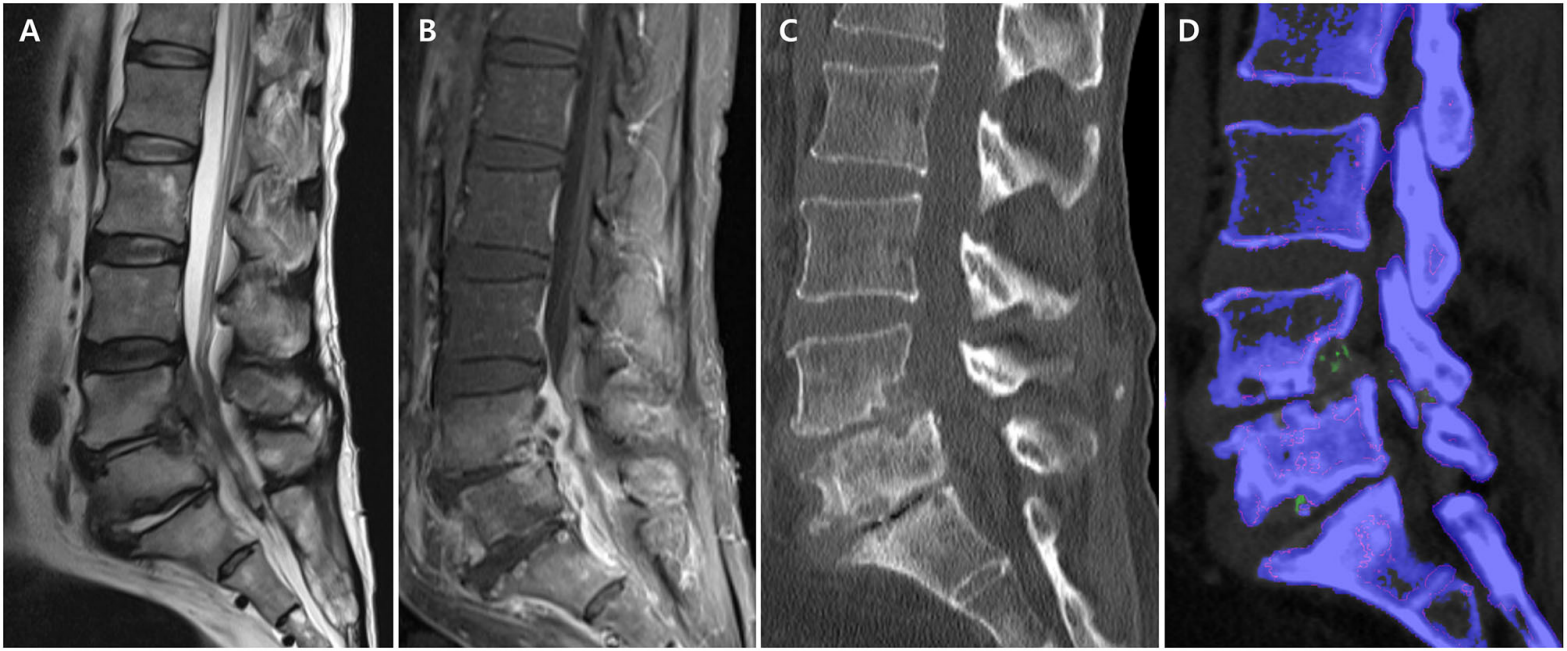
Lumbar spine magnetic resonance imaging and computed tomography (CT) in axial gout. (A) Increased signal intensity on T2WI of L4-5 and L5-S1 intervertebral disc and erosive changes on the posterior cortices and endplates of L4-L5-S1 vertebra. (B) Enhancement of epidural space on T1WI. (C) Erosive changes in L4-5 and L5-S1 endplates on conventional CT. (D) Monosodium urate deposits (green) in the erosive foci of endplate on dual-energy CT.
